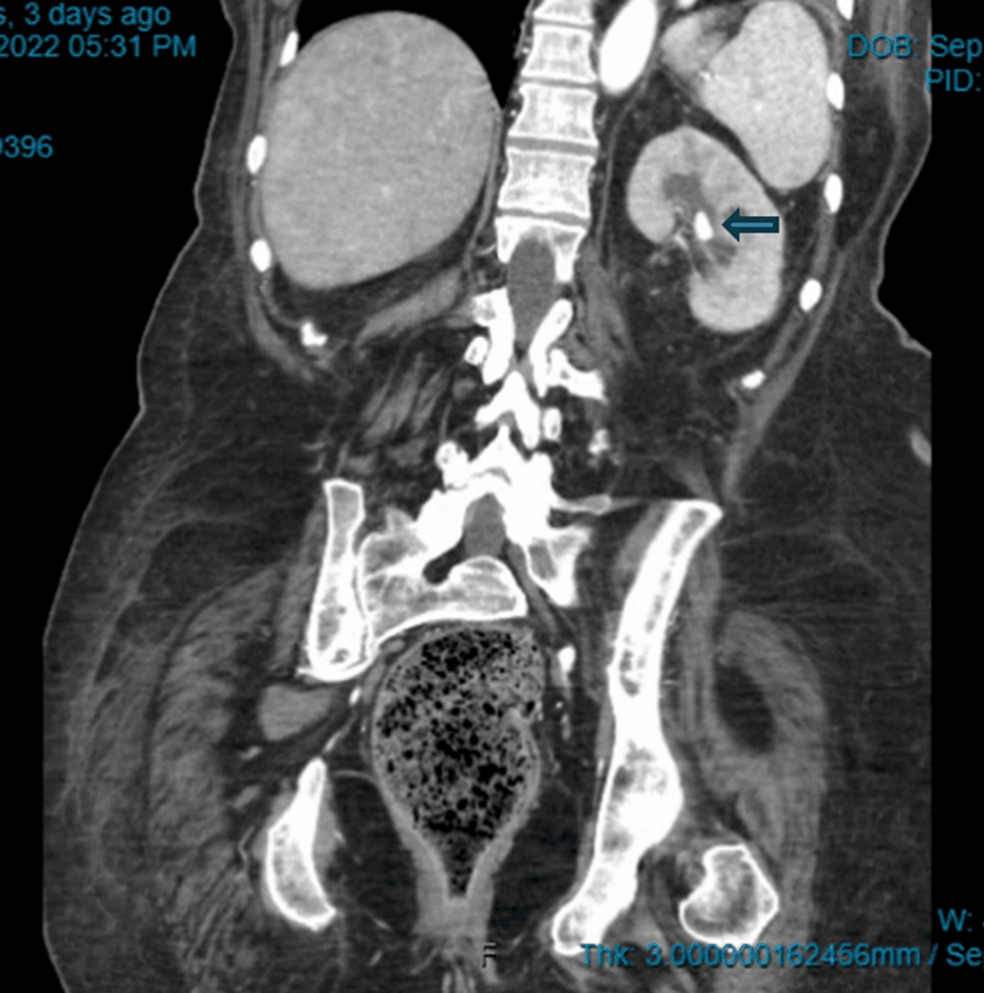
Coronal view CT scan of the abdomen and pelvis showing left-sided nephrolithiasis The blue arrow demonstrates a calcified stone measuring 11 × 11 × 10.4 cm.
